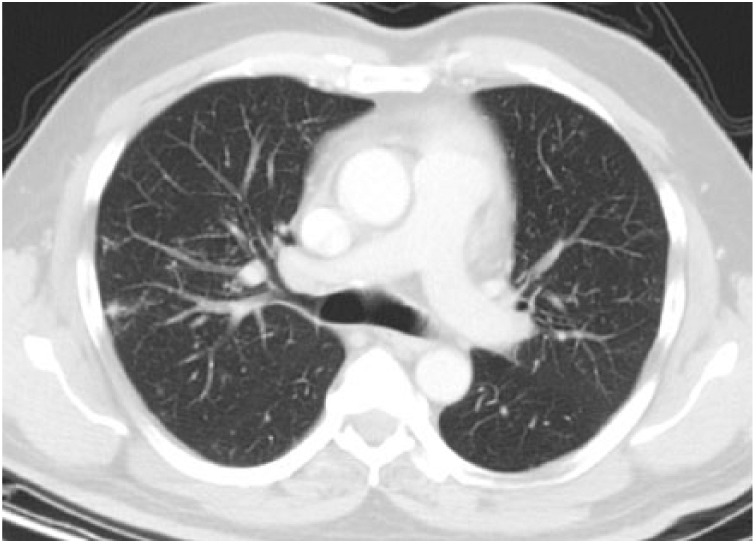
Control thorax HRCT parenchymal window showing regression of nodules and interstitial shadows.
